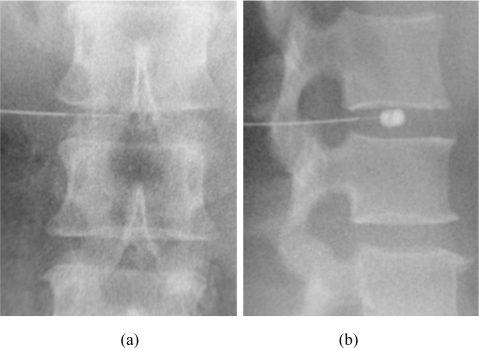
Needle placement for lumbar discography. (a) Anteroposterior and (b) lateral radiographic projections show the tip of the thicker, shorter outer needle at the posterolateral corner of the annulus fibrosis of the intervertebral disc. The tip of the thinner, longer inner needle is located in the centre of the intervertebral disc. Test injection of contrast agent confirms that the inner needle tip lies in the nucleus pulposus.
